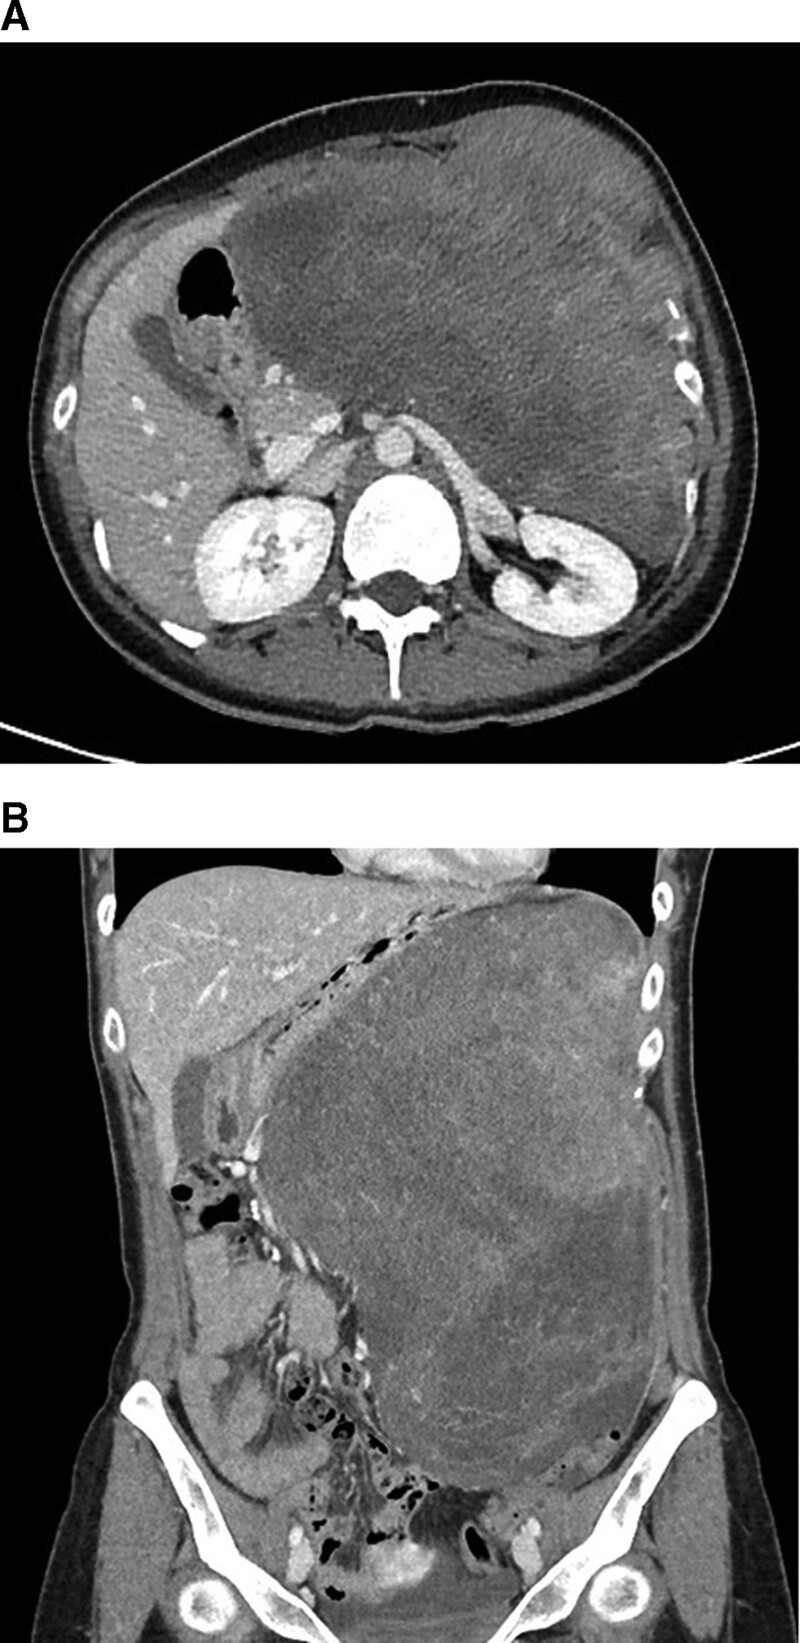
Contrast-enhanced abdominal computed tomography. (A) Transverse view of contrast-enhanced abdominal CT. (B) Coronal view of contrast-enhanced abdominal CT. About 29 × 17cm heterogeneous soft tissue mass in left abdomen with invasion to left anterior abdominal wall and costochondral junction of left 8th to 10th ribs resulting pathological fracture.
